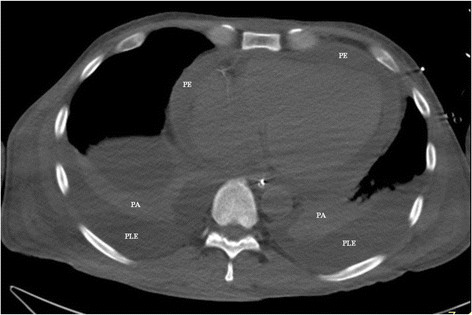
CT scanning of the thorax without contrast. The pericardial effusion (PE) surrounding the heart is about 15 mm thick. PLE, bilateral pleural effusions; PA, pulmonary atelectasis.
